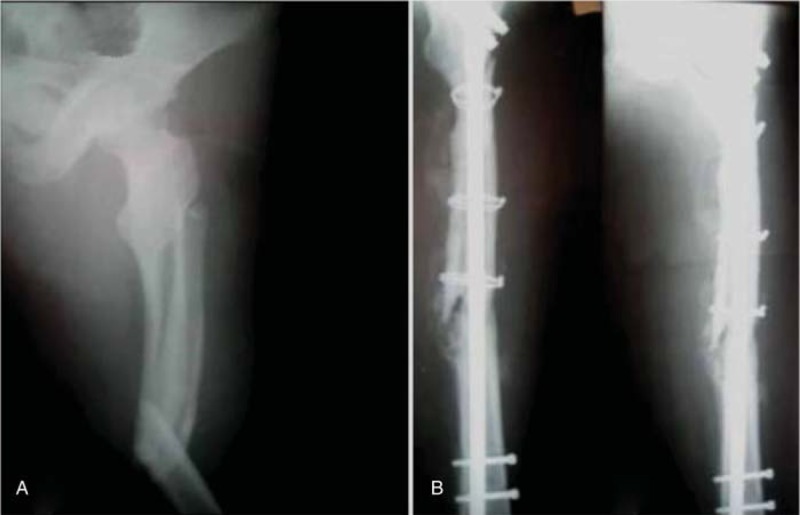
A, Radiograph of a 18-year-old man (case 6) who had an middle femoral shaft fracture. B, Anterioposterior and lateral X-ray of femora after performing the operation of IM nailing merging. IM = intramedullary.
